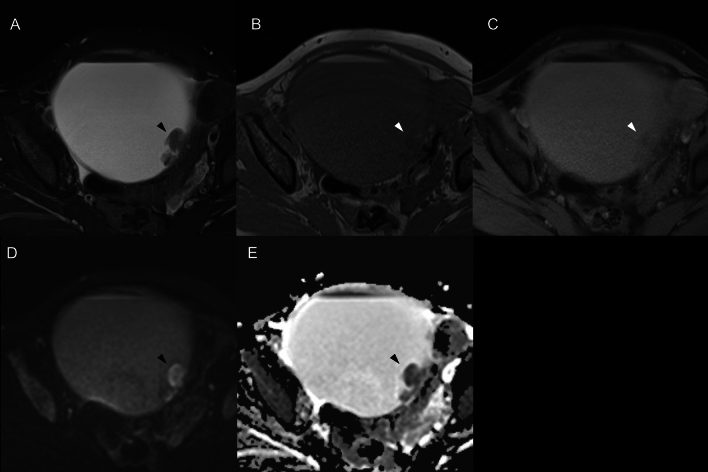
A 47-year-old woman with squamous cell carcinoma arising in an ovarian cystic teratoma without transmural extension. MR images reveal mural nodule consistent with “findings suggestive of malignancy” (mural nodule with diffusion restriction and without fat content, calcification, and Palm tree appearance). a Axial fat-suppressed T2-weighted image shows a 155 mm-sized large cystic lesion in the pelvis with typical fat-fluid level. The mural nodule (arrowhead) is located on the left side of cystic wall, forming an acute angle with the cystic wall and does not exhibit the Palm tree appearance. b Axial T1-weighted in-phase image could not demonstrate the mural nodule clearly. c Axial T1-weighted fat-suppressed images do not show the signal drop of the nodule. The solid nodule does not exhibit any strong hypointense areas suggestive of calcification. d, e The mural nodule shows hyperintensity on diffusion-weighted images and hypointensity on apparent diffusion coefficient maps, suggesting restricted diffusion
